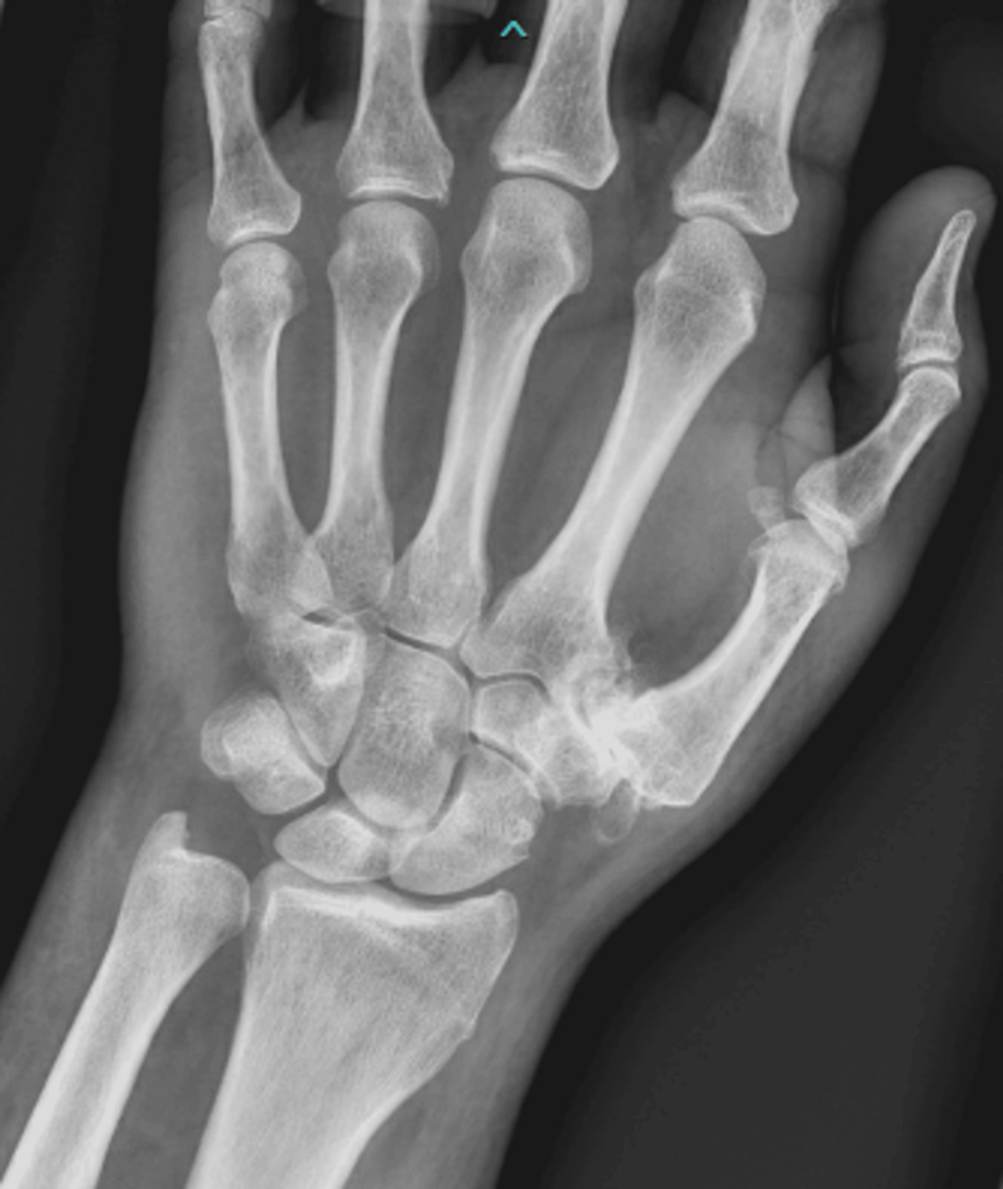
Initial left-hand radiograph.
